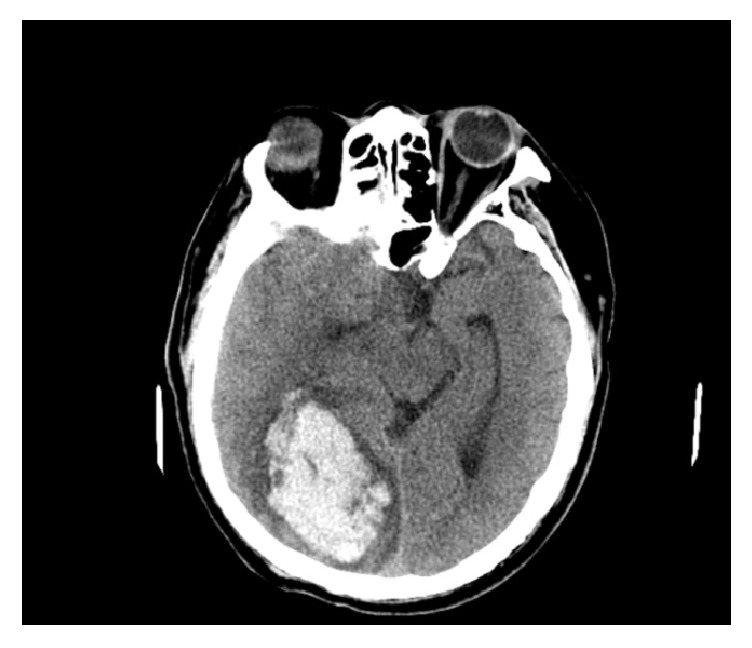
Noncontrast head CT performed on the 7th day demonstrates an acute hemorrhage in the right occipital lobe, with surrounding oedema and compressing the right lateral ventricle with midline shift to left.
